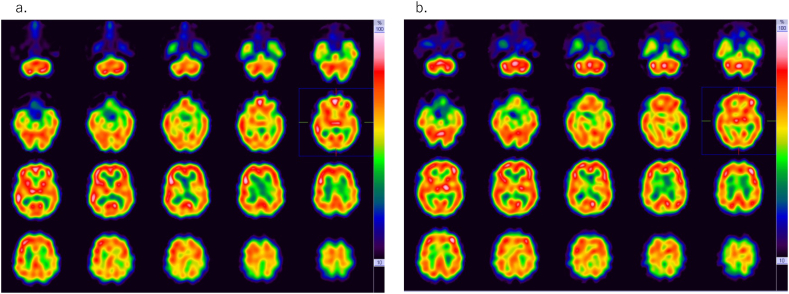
IMP-SPECT before (a) and after (b) CEA in case 2 Compared with preoperative SPECT, postoperative SPECT (b) shows a 5%–10% increase in the cerebral blood flow in the left MCA territory (a). SPECT: single-photon emission computed tomography, IMP-SPECT: N-isopropyl-p-[123I]-iodoamphetamine SPECT, CEA: carotid endarterectomy, MCA: middle cerebral artery.
